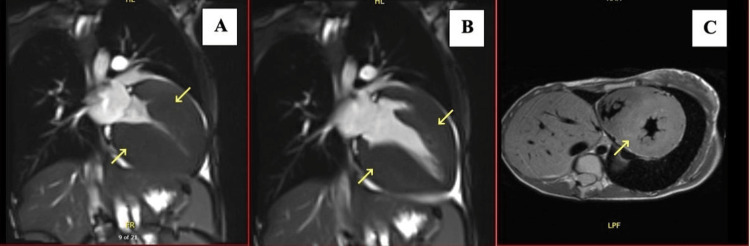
Cardiac magnetic resonance imaging (CMRI) Two-chamber view showing the left ventricle (LV) during systole through a balanced steady-state free-precession (bSSFP) (4A); two-chamber view showing LV during diastole through a bSSFP (4B); T2-weighted short axis view showing both ventricles (4C)
